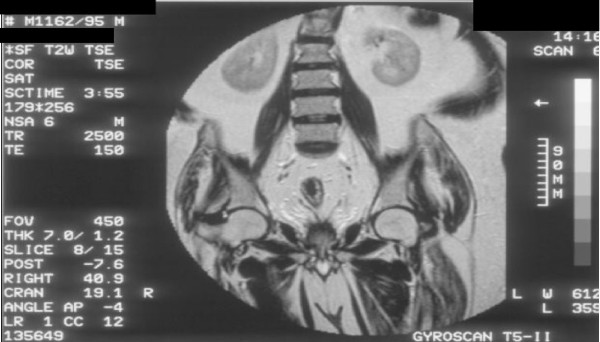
Magnetic Resonance Imaging of pelvis performed on 23 October 1995: Coronal T-2 weighted image shows no extra-capsular spread of carcinoma of prostate. Seminal vesicles appear normal.
